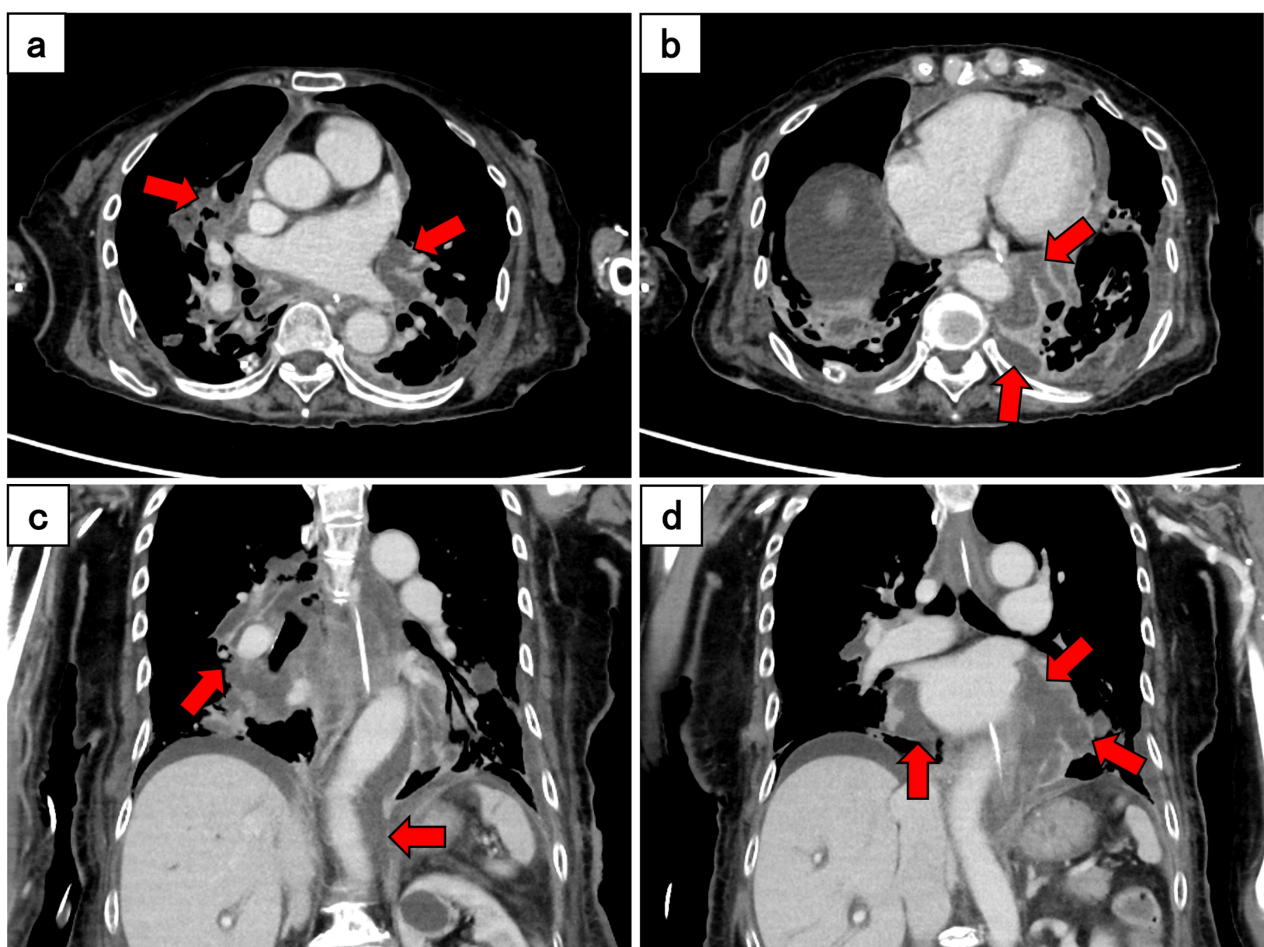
Contrast-enhanced computed tomography on postoperative day 16 in the axial (a and b) and coronal (c and d) planes Expanding low-density areas (red arrows) around the aorta and bilateral hilar vessels indicate abscess progression. On the right side, low-density areas extend to the superior mediastinum and interlobar fissures (upper-lower and middle-lower). The infection involves the vascular sheaths of the pulmonary arteries and veins. On the left side, low-density areas were present around the inferior pulmonary vein and the lung base. Aortic involvement extended to the diaphragmatic crura (red arrows). A small amount of ascitic fluid was also observed.
